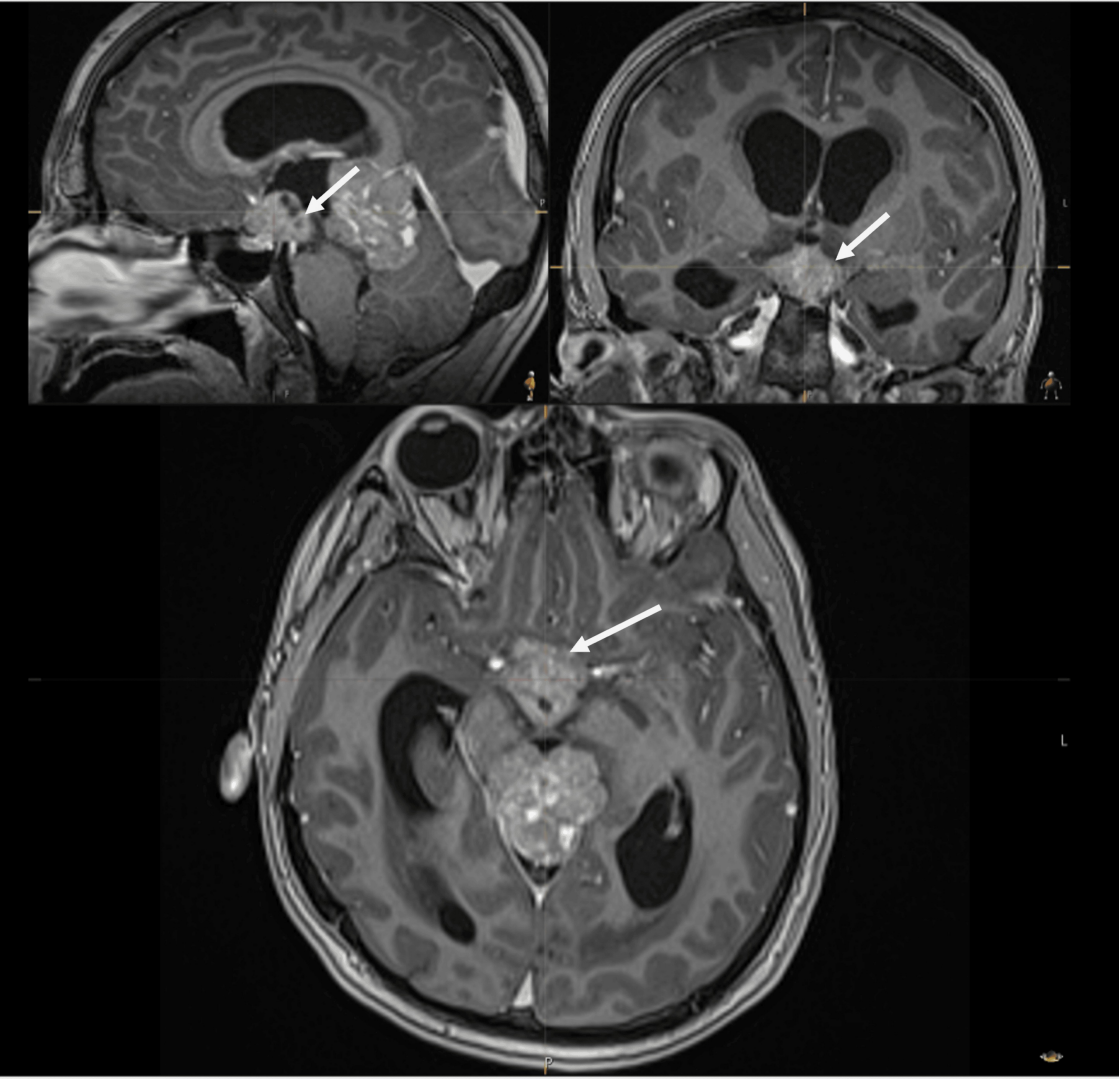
MRI of the head with contrast. White arrows: mass in the suprasellar region.
